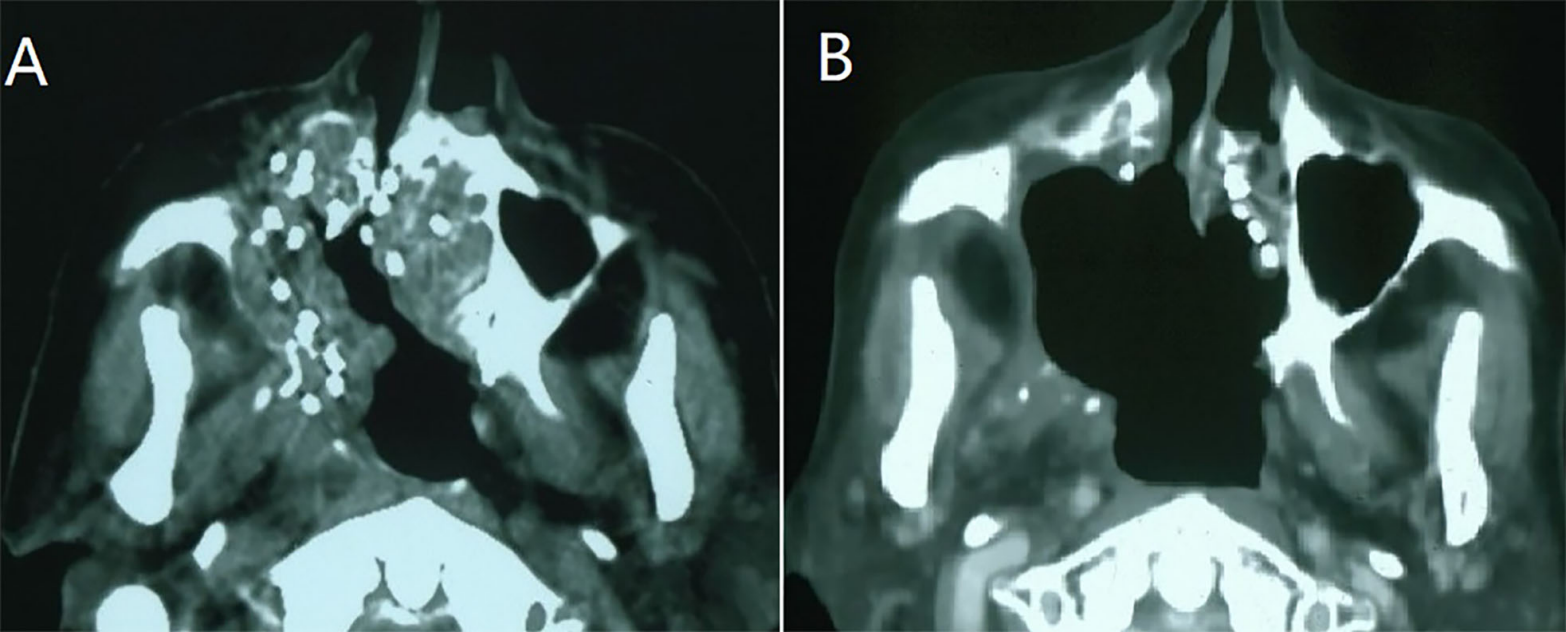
A case of recurrent maxillary sinus squamous carcinoma following surgery and adjuvant radiotherapy. (A) CT-guided I^125^ seeds were implanted as a salvage treatment; (B): Three months after the seed implantation, partial response was observed.
